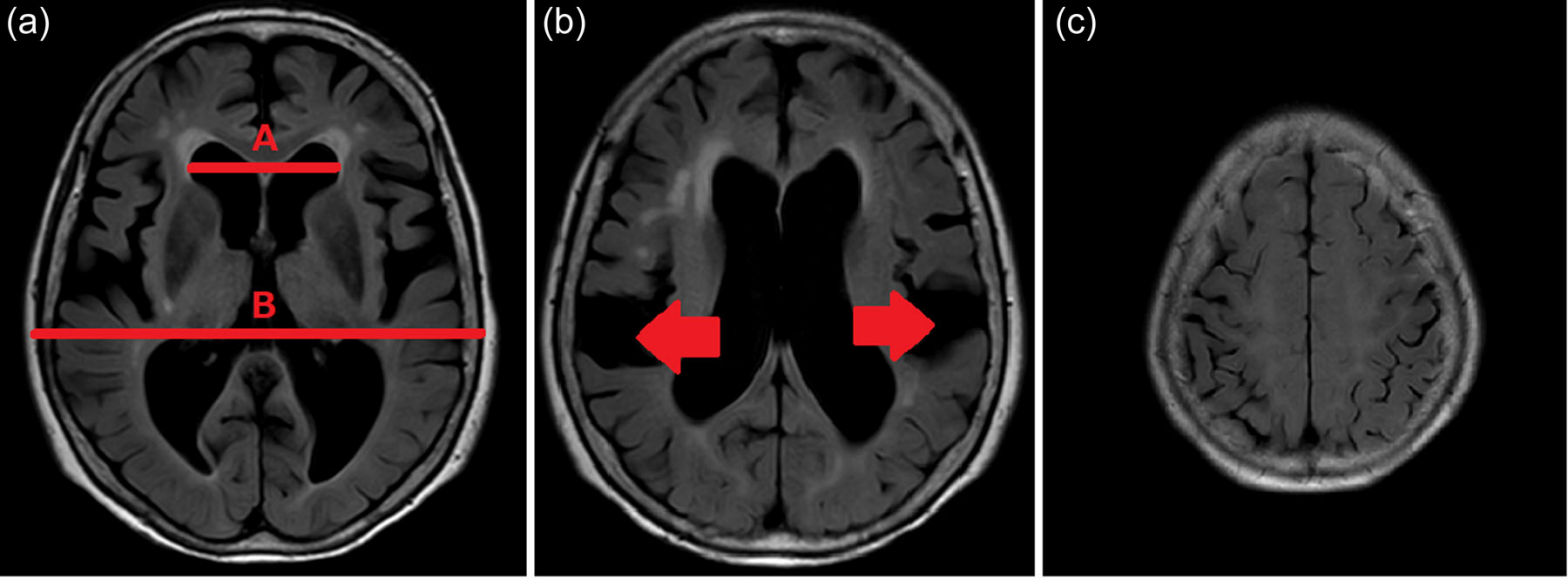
Brain magnetic resonance imaging (MRI). MRI showed enlargement of the ventricles (red lines in A and B) and subarachnoid space below the Sylvian fissure (B, red arrows), and a narrowing at the higher arcuate region (C).
